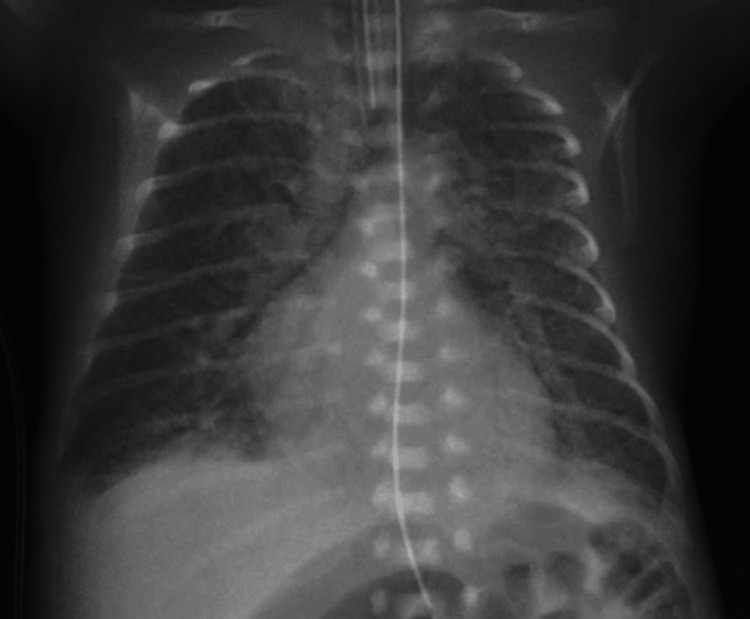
Chest X-ray at 10 days of life The chest X-ray shows irregular opacities in both lung fields, and the borders of the heart and the diaphragm are obscured
